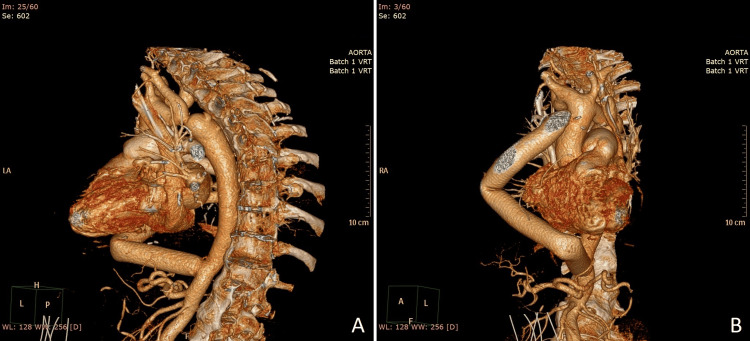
Postoperative CT angiography demonstrating the patency of the extra-anatomic bypass. (A) View of the distal anastomosis. (B) View of the proximal anastomosis.
